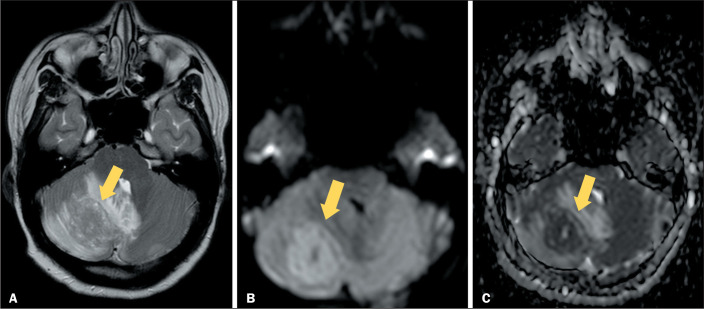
MRI of an 18-year-old patient diagnosed with mediastinal non-Hodgkin
